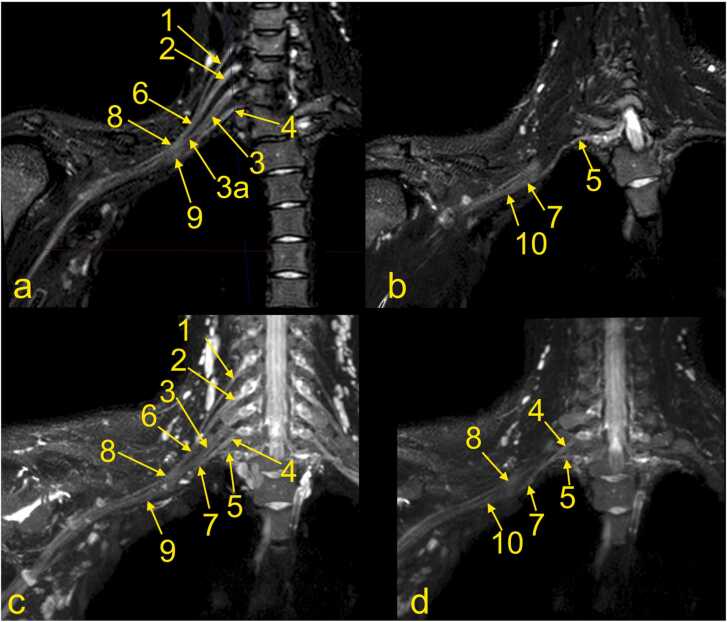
MRI of the brachial plexus, right side. (a and b) T2-weighted DIXON sequence with fat suppression. (b) MPR of the C8 root. (c and d) Neurography. 1 – the C5 root, 2 – the C6 root, 3 – the middle trunk, 4 – the C8 root, 5 – the Th1 root, 6 – the upper trunk, 7 – the lower trunk, 8 – the lateral cord, 9 – the medial cord, and 10 – the posterior cord.
